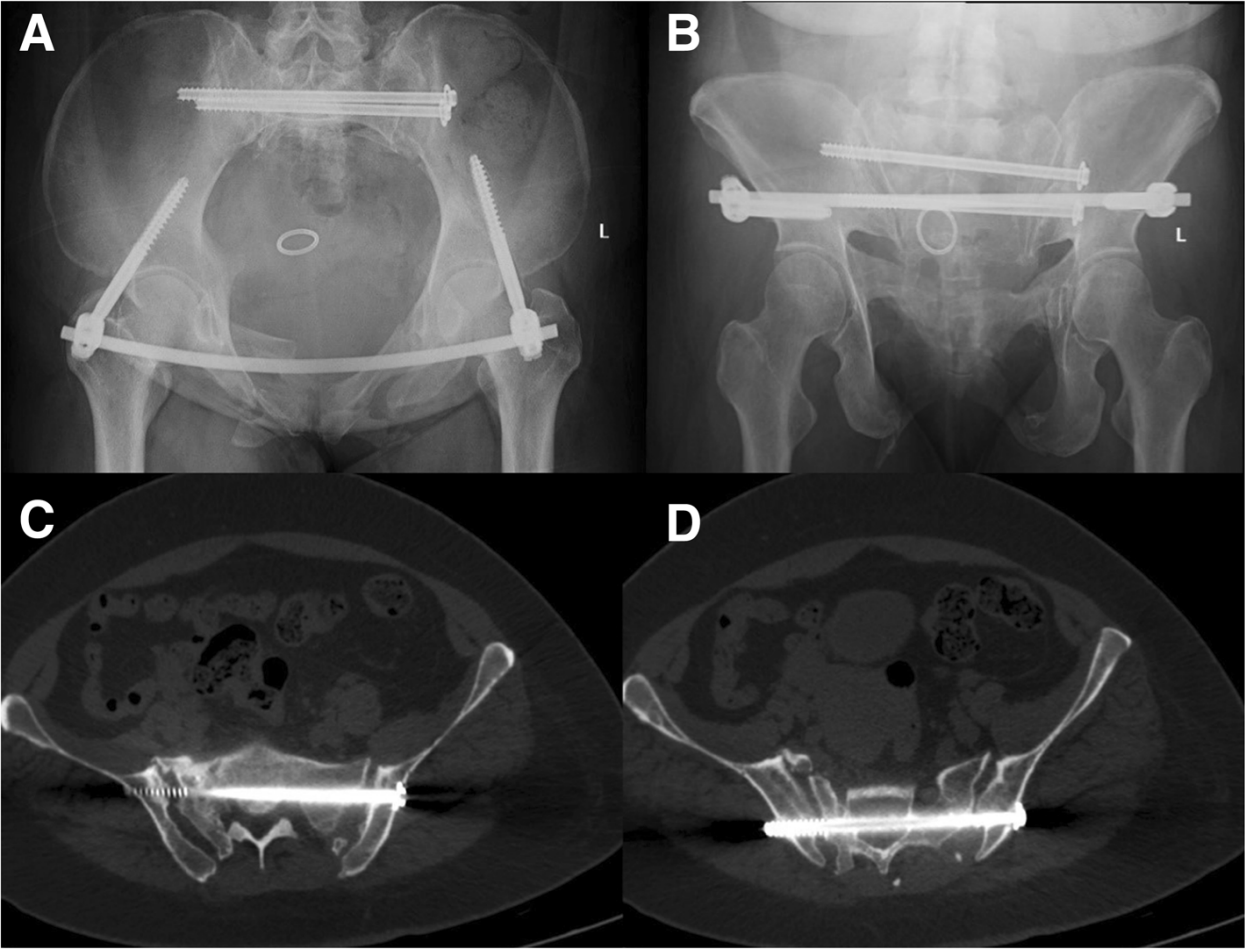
The inlet and outlet views and CT scan of the pelvis at 2 years of follow-up showed good position of screws and fracture healed. a, b Inlet and outlet views of the pelvis. c, d S1 screw and S2 screw positions of CT scan
